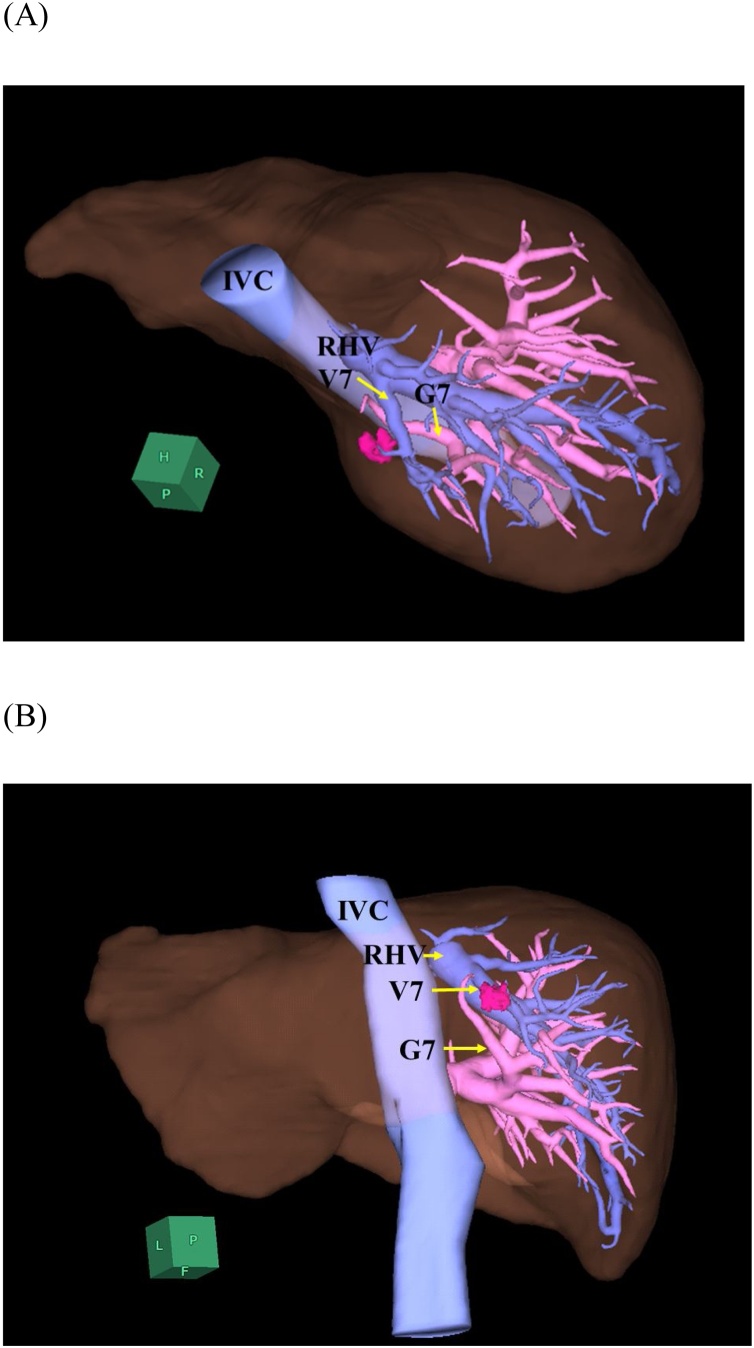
The three-dimensional imaging based on computed tomography showed colorectal liver metastasis, located at the top of segment 7 behind the right hepatic vein. IVC, inferior vena cava; RHV, right hepatic vein; G7, Glissonean pedicle of segment 7; and V7, venous branch of segment 7.
